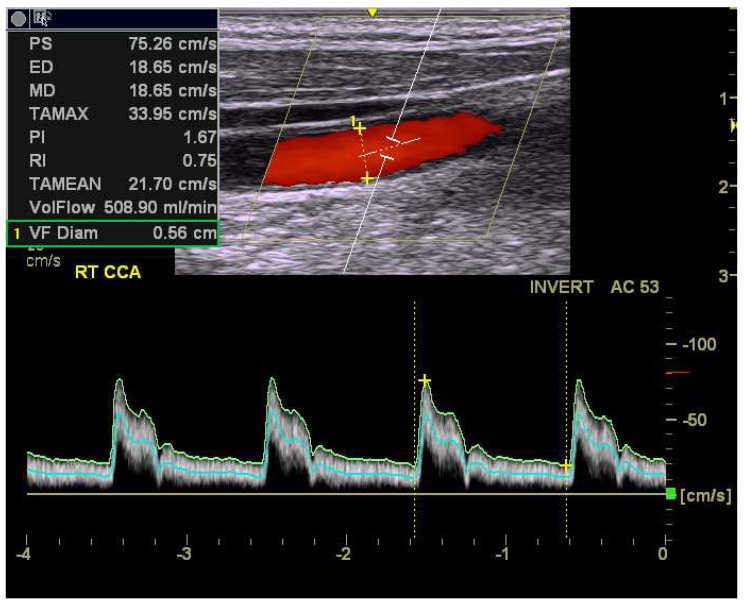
A representative Doppler image of the right CCA.
